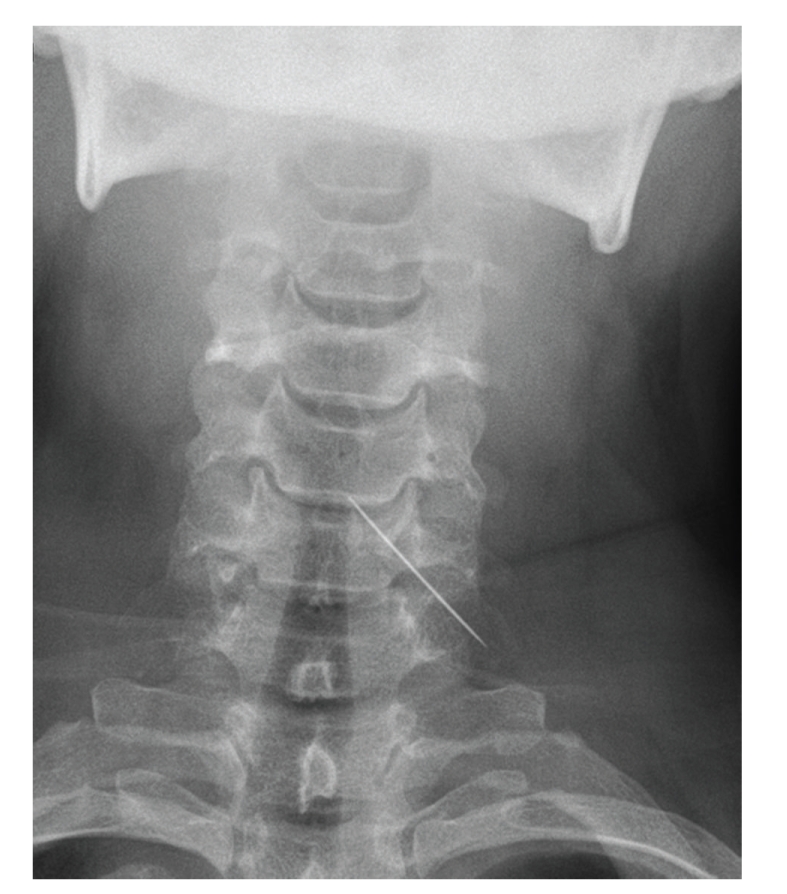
Postero-anterior cervical spine radiograph.
